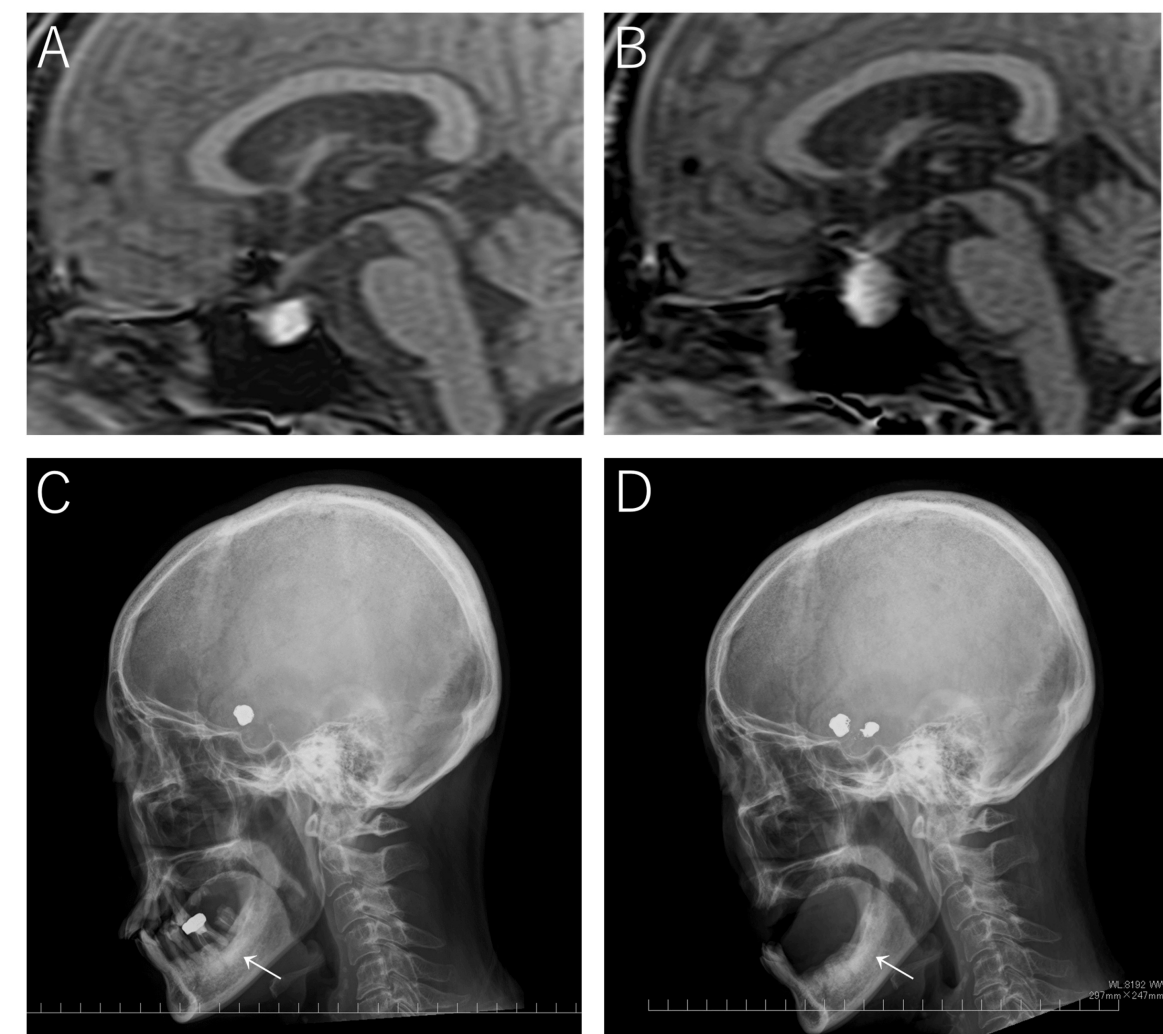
(A) Sagittal view of the magnetic resonance image showing that the pituitary gland is enlarged at postoperative month (POM) 1. (B) The size of the pituitary gland is dramatically increased at POM 7. (C) Lateral view of the cranial radiography image showing that most of the teeth were intact when the aneurysms were treated. (D) Almost all of the teeth had been extracted when the pituitary abscess was treated.
